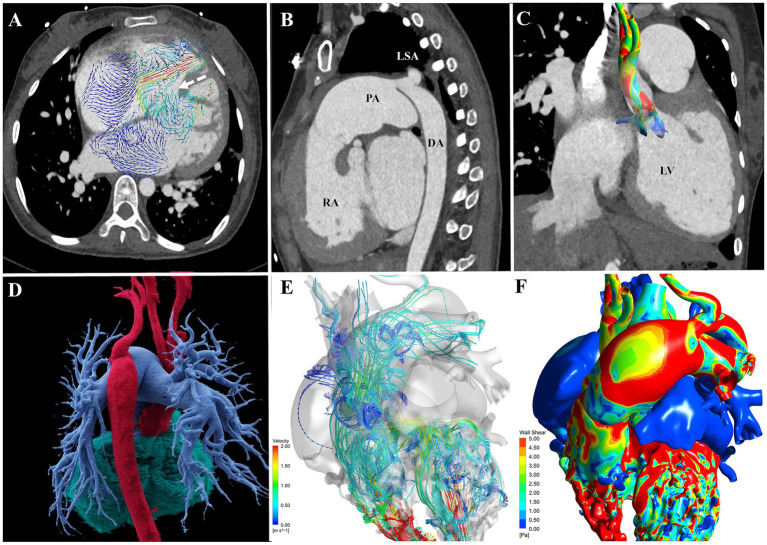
Four-dimensional cardiac CT and computational fluid dynamics of type B interrupted aortic arch (IAA) with a ventricular septal defect (VSD) and patent ductus arteriosus (PDA). (A) Axial (four-chamber) view showing the ventricular septal defect (white dashed arrow) with abnormal flow across the interventricular septum on color Doppler. (B) CT sagittal view showing the thoracic aorta originating from the right pulmonary artery, with its distal segment supplied by the left subclavian artery. (C) CT oblique coronal view fitted with hemodynamic overlays showing the right common carotid, left common carotid, and right subclavian arteries arising from the aortic trunk, together with wall shear stress (WSS). (D) Cinematic rendering highlighting the thoracic aorta arising from the right pulmonary artery, with its distal segment supplying the left subclavian artery. (E) Because of the ventricular septal defect, blood flow between the left and right ventricles created a communication, with most of the blood flow directed towards the pulmonary artery (bidirectional, predominantly left-to-right). (F) Wall shear stress (WSS) analysis indicated that the overall WSS in the pulmonary artery was higher than that in the aorta in this case. PA, pulmonary artery; Ao, aorta; PDA, patent ductus arteriosus; VSD, ventricular septal defect; WSS, wall shear stress.
